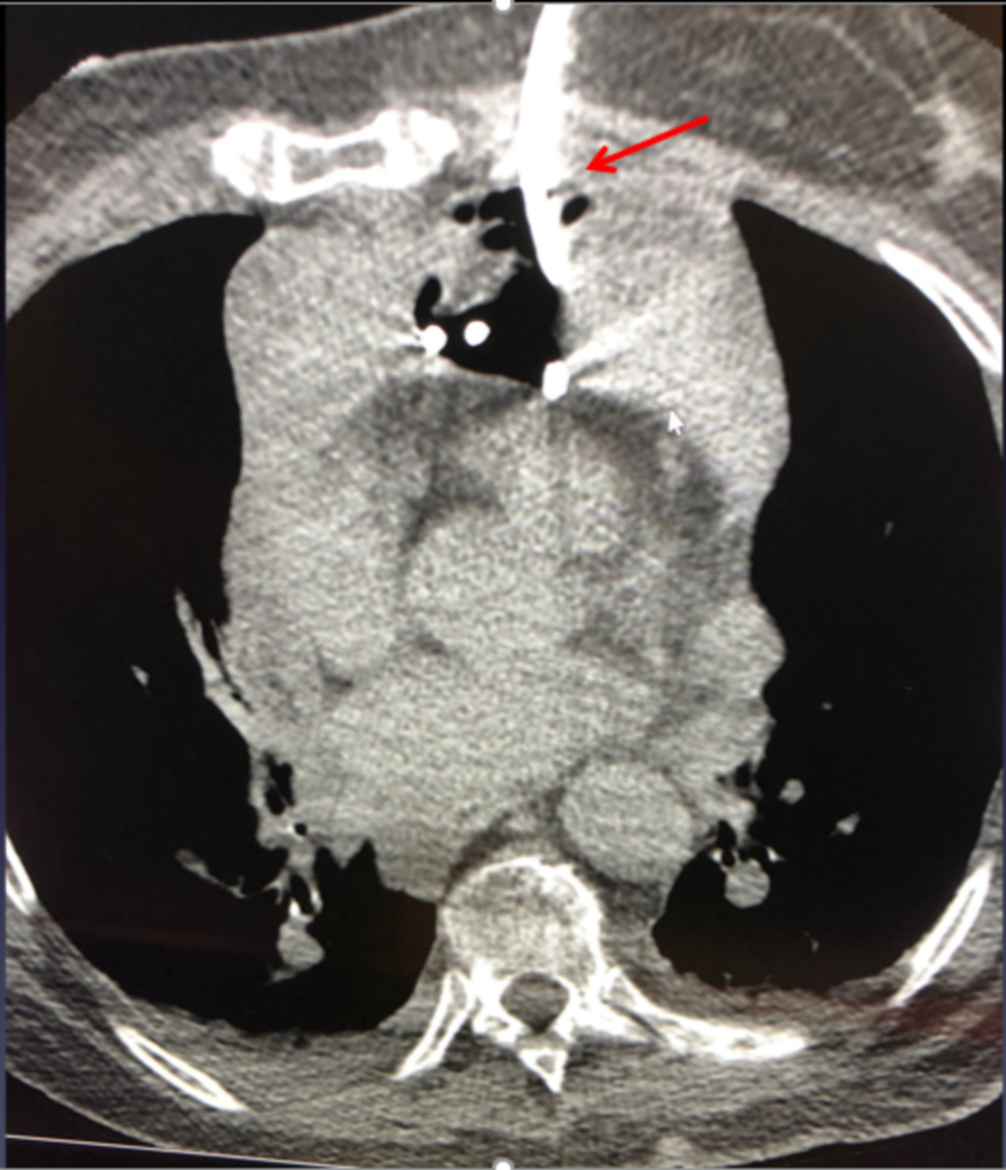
CT-guided drainage of mediastinal hematoma with an 8.5-French pigtail catheter.
